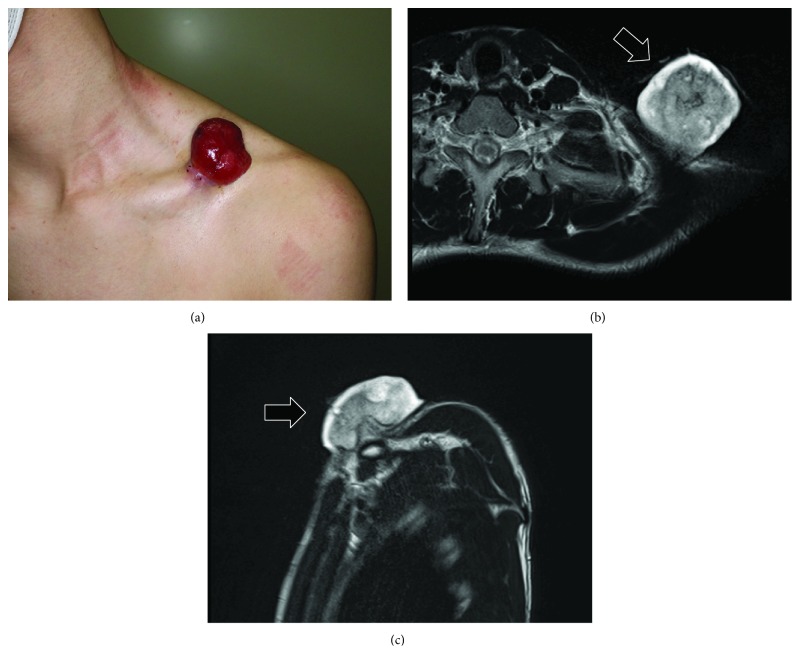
(a) Frontal view of the large protruding tumor, located on the anterior chest wall, measuring 4 × 3 cm at the first visit. (b, c) Axial (b) and sagittal (c) view of the tumor (arrow) on T2-weighted magnetic resonance imaging.
